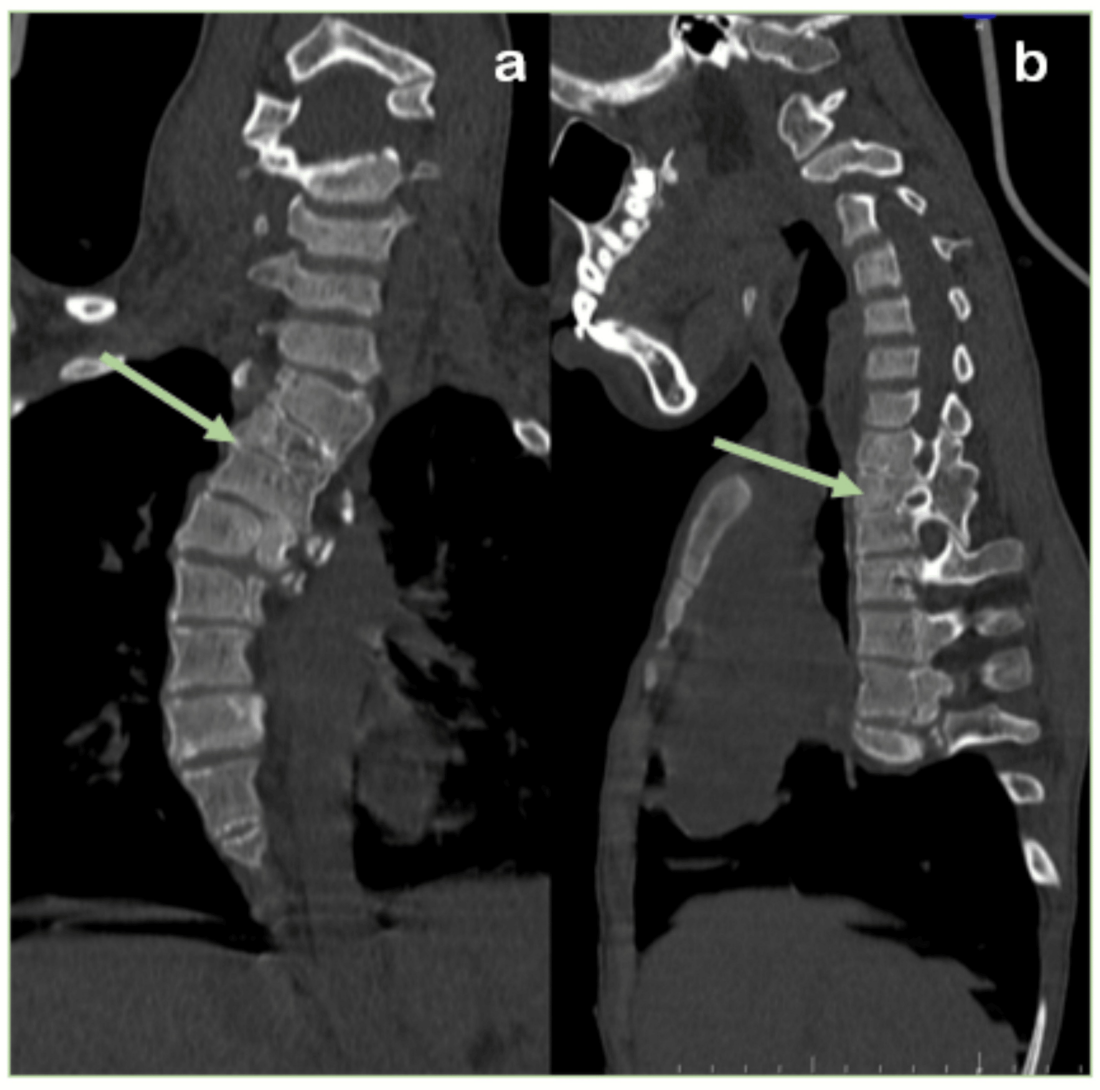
Computed tomography: (a) spine coronal and (b) sagittal images show congenital dorsal block vertebrae (green arrows).
